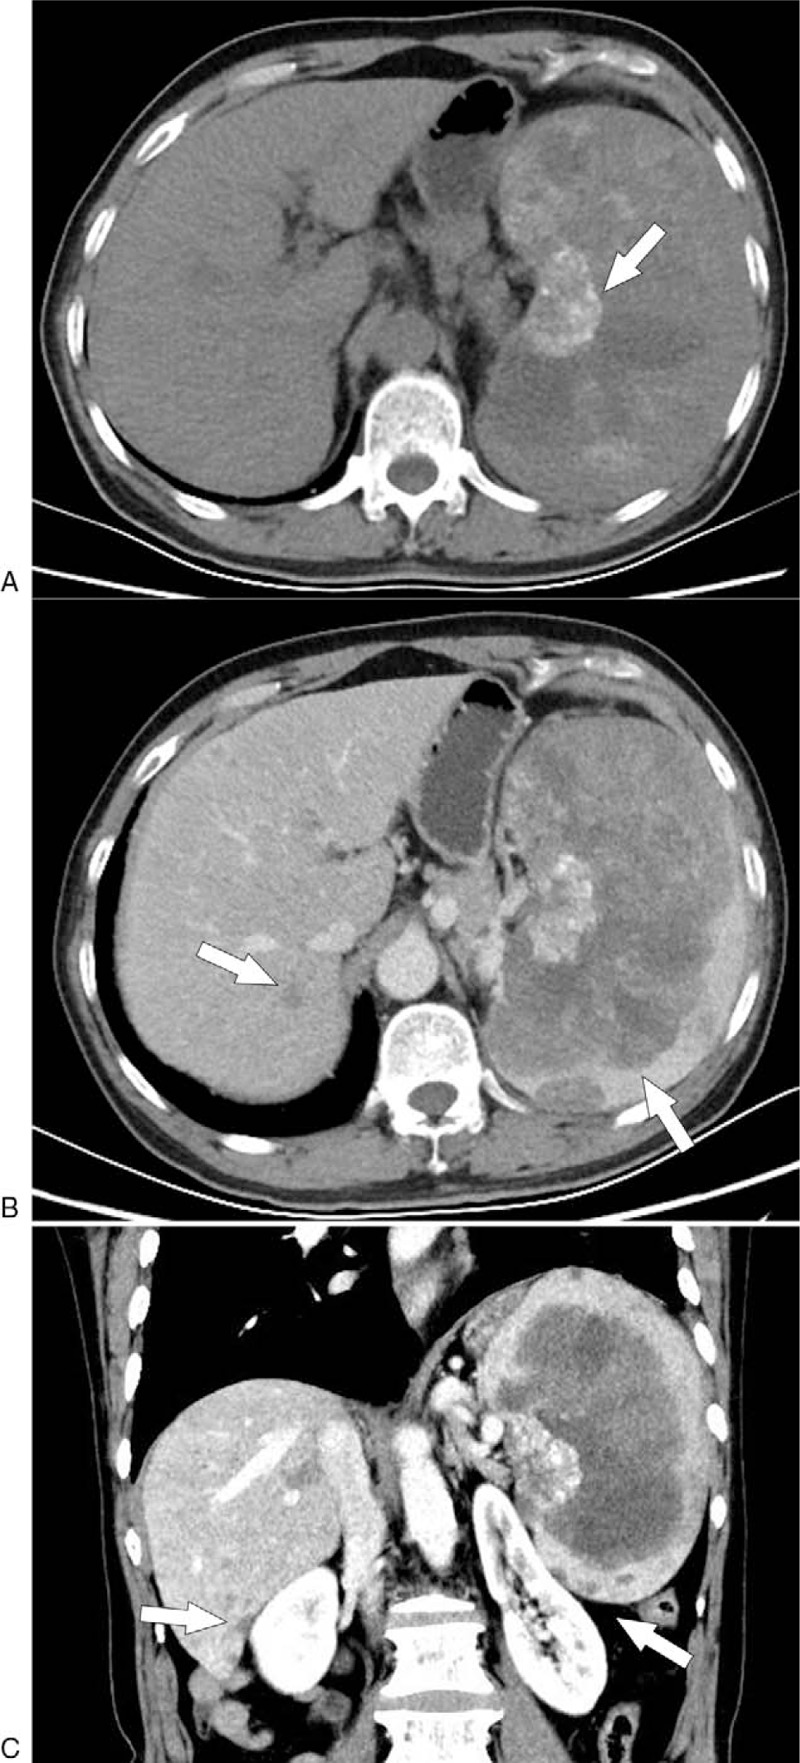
Axial: Unenhanced CT scan of the abdomen shows mixed density image with unclear boundary and cloud-like calcification in enlarged spleen (arrow) (A). Enhanced CT showed multiple slightly heterogeneous enhancement of the lesions in the spleen, and the boundary of the tumor was well-defined in the venous phase (arrows) (B). Coronal: In the venous phase, the liver is detected with multiple spotty lesions with slightly enhanced and left kidney is compressed (arrow) (C).
